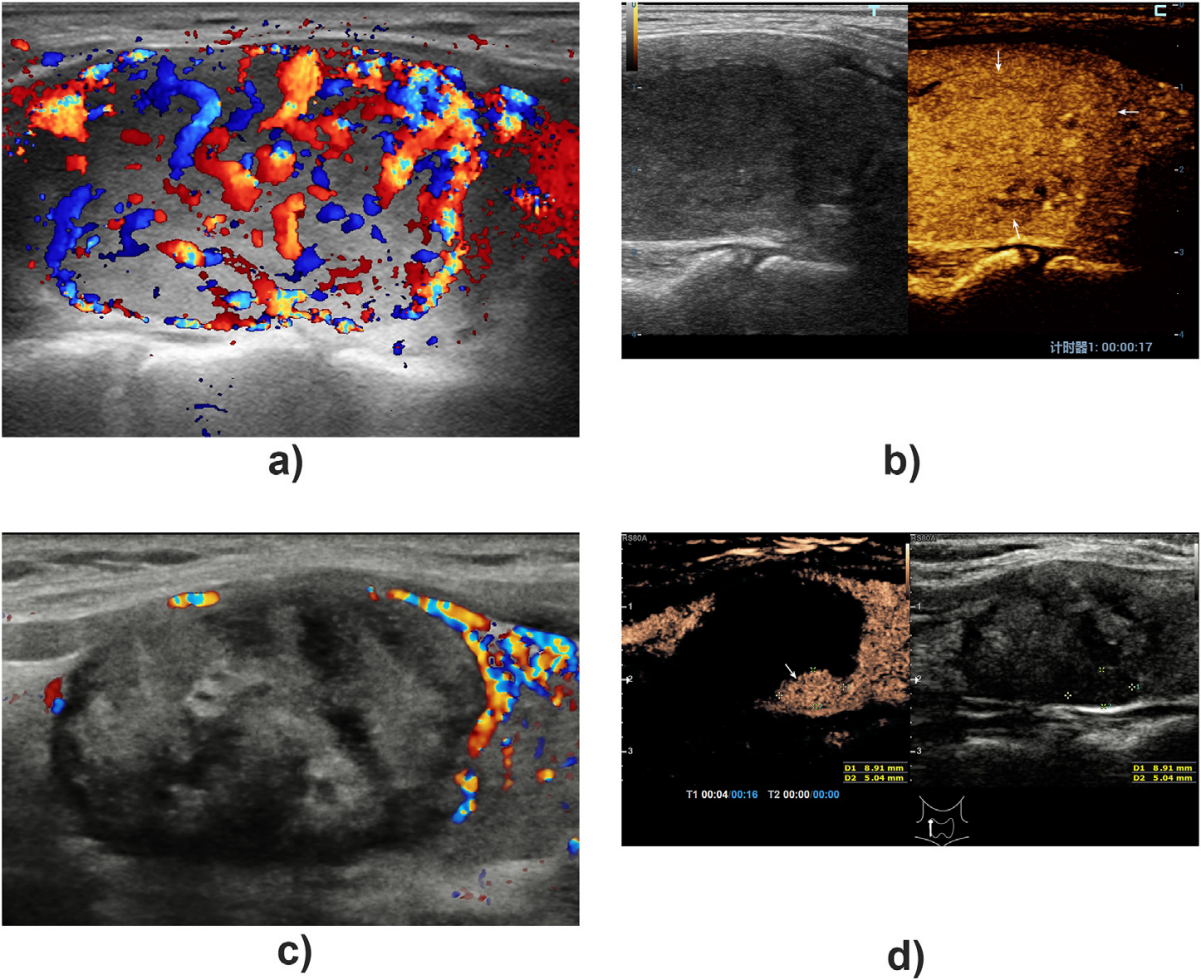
A re-treat BTN. A 55-year-old woman with a benign nodule in her right lobe of thyroid for 12 years follow-up. Abundant flow signals could be detected inside and around the nodule on color flow image (A). On contrast-enhanced ultrasound, the nodule showed uniform iso-enhancement (arrow) in arterial phase before microwave ablation treatment (B)·In follow-up after 6 months, conventional ultrasound reveal a heterogenous hypoechoic nodule without inside color flow signals (C). However, a small nodule-like enhancement area (arrow) was found in the deep position of the ablation area during contrast-enhanced ultrasound (D).
